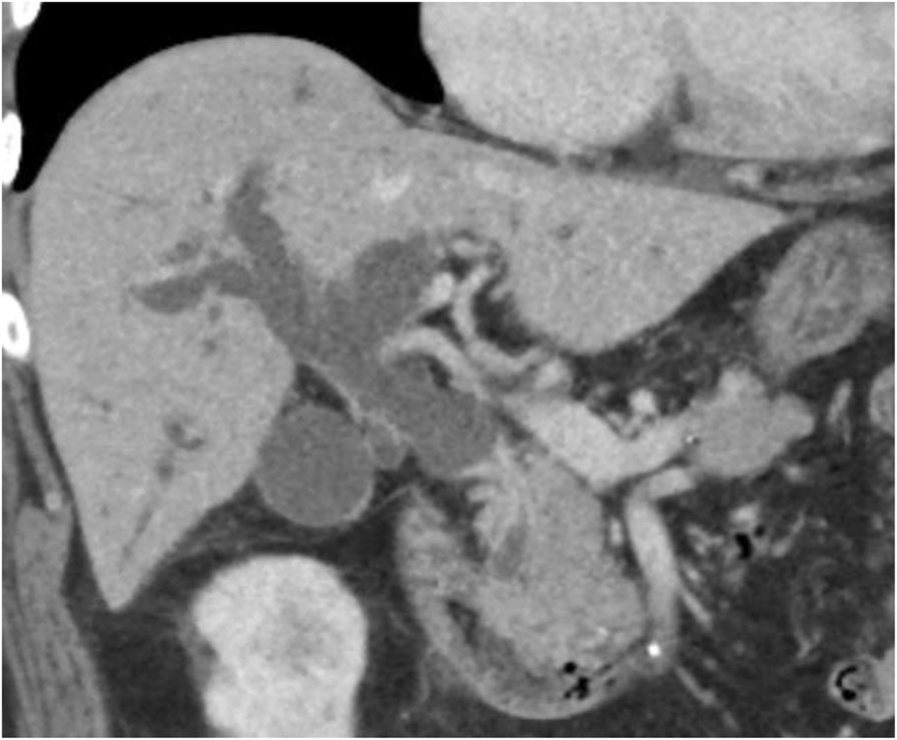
Three-dimensional computed tomography showing a common bile duct tumor
